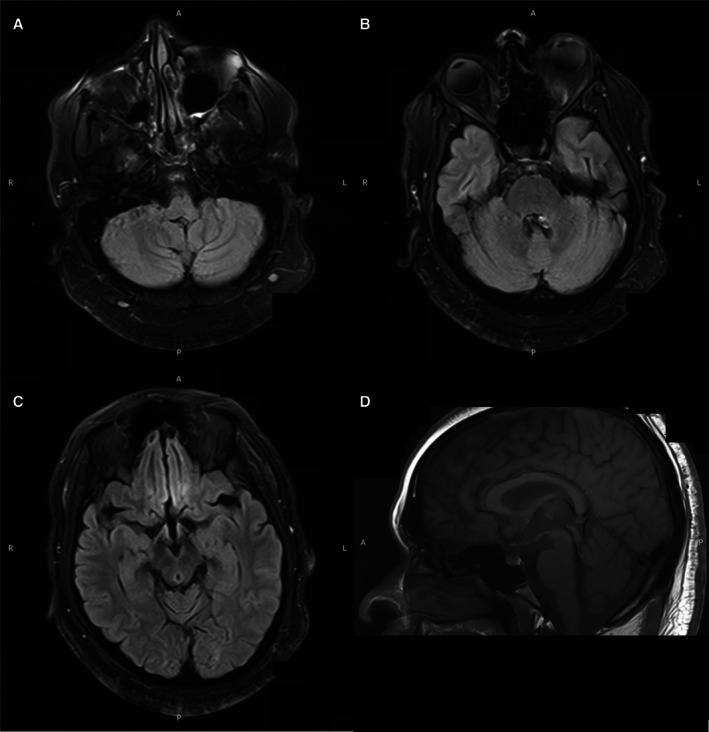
Axial FLAIR (A–C) and sagittal T1‐weighted (D) brain MRI demonstrating no structural abnormalities.
